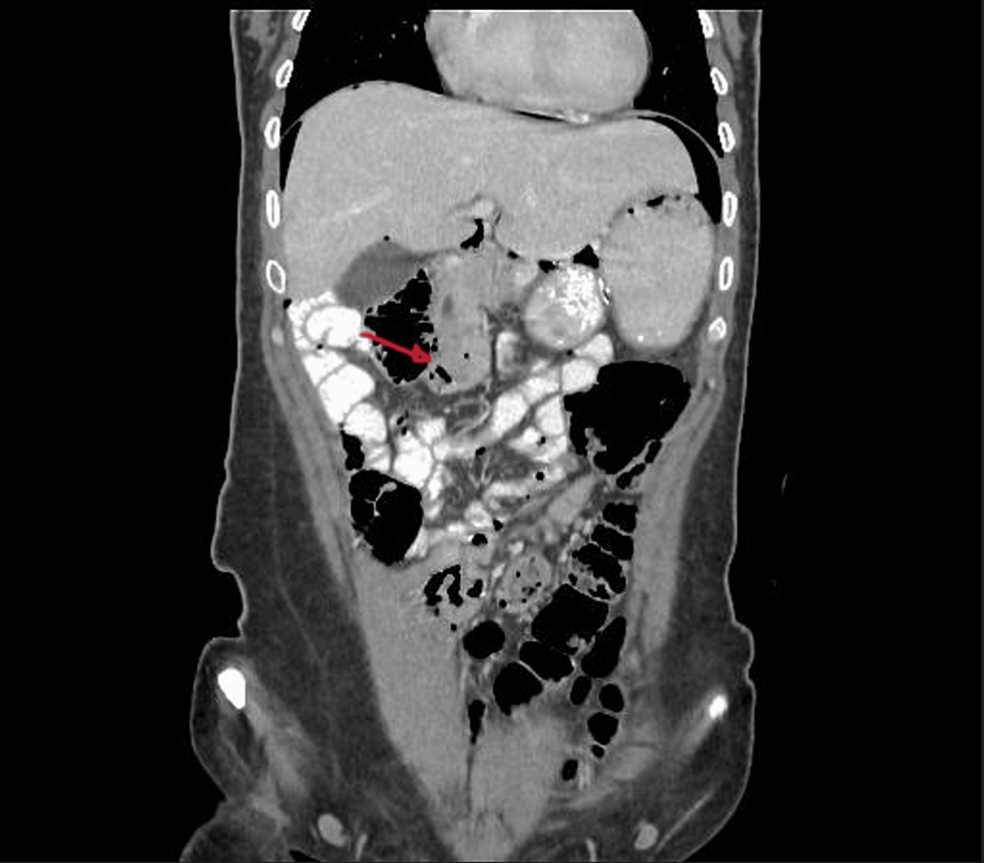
CT of the abdomen showing pneumoperitoneum and duodenojejunal junction (red arrow) observed on the right side of the abdomen
